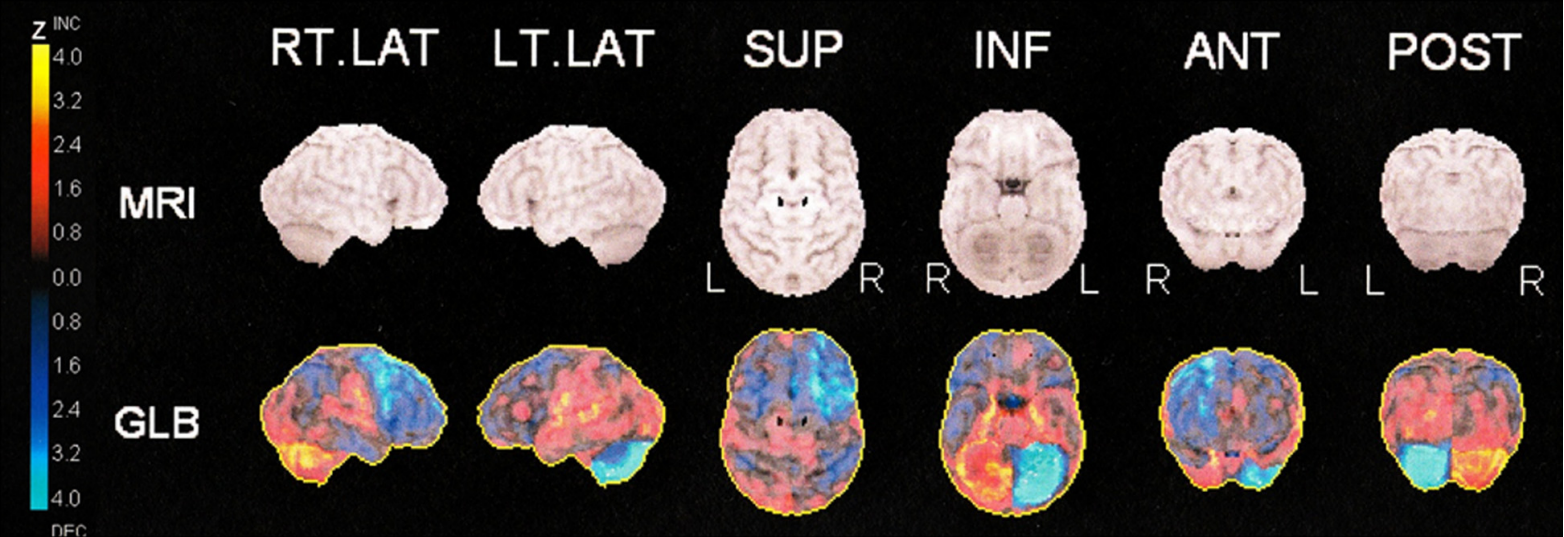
Quantitative 3-dimensional surface projection-analysis of FDG-uptake in patient no 25. PET-images of patient no. 25 are normalized to whole brain using a database of normal subjects and scaled to Z values from - 4.0 to 4.0 (Neurostat). Projections of Z values are shown onto, respectively, the right and left lateral hemispheric surfaces, the superior and inferior surfaces, and the anterior and posterior surfaces. The uptake in the left cerebellar hemisphere is reduced in addition to uptake in the right frontal cortex. MRI surface projections are presented for anatomical reference in a standard stereotactic space
